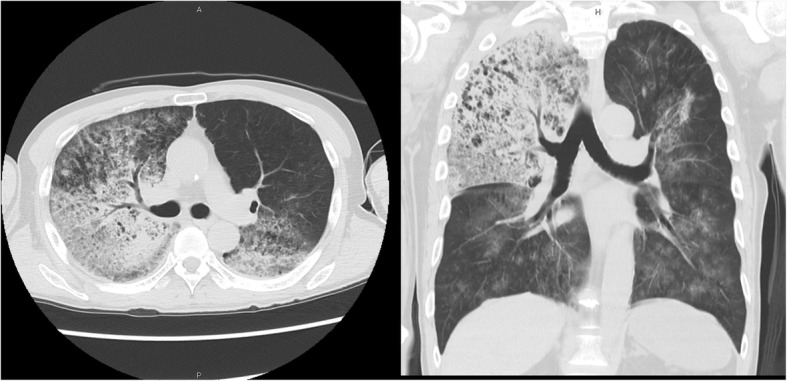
Chest CT showing infiltration predominantly in the right upper lobe and spreading to the right middle and lower lobe and left hilar area, suggesting alveolar hemorrhage
